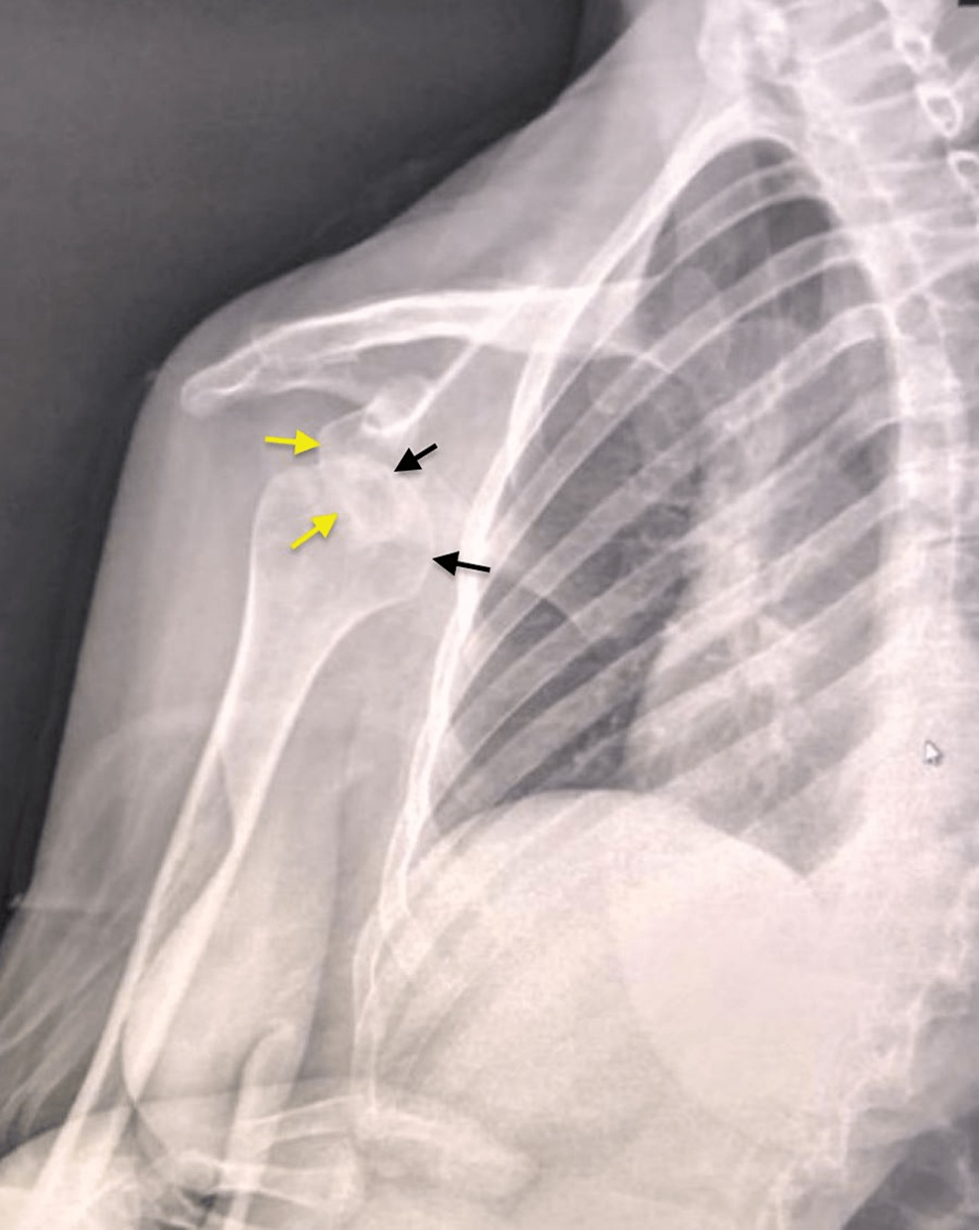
Frontal preoperative X-ray showing anterior dislocation of the right shoulder since three months in a 30-year-old patient. Also seen is the contact loss between the humeral head (black arrows) and shoulder blade glenoid cavity (yellow arrows).
